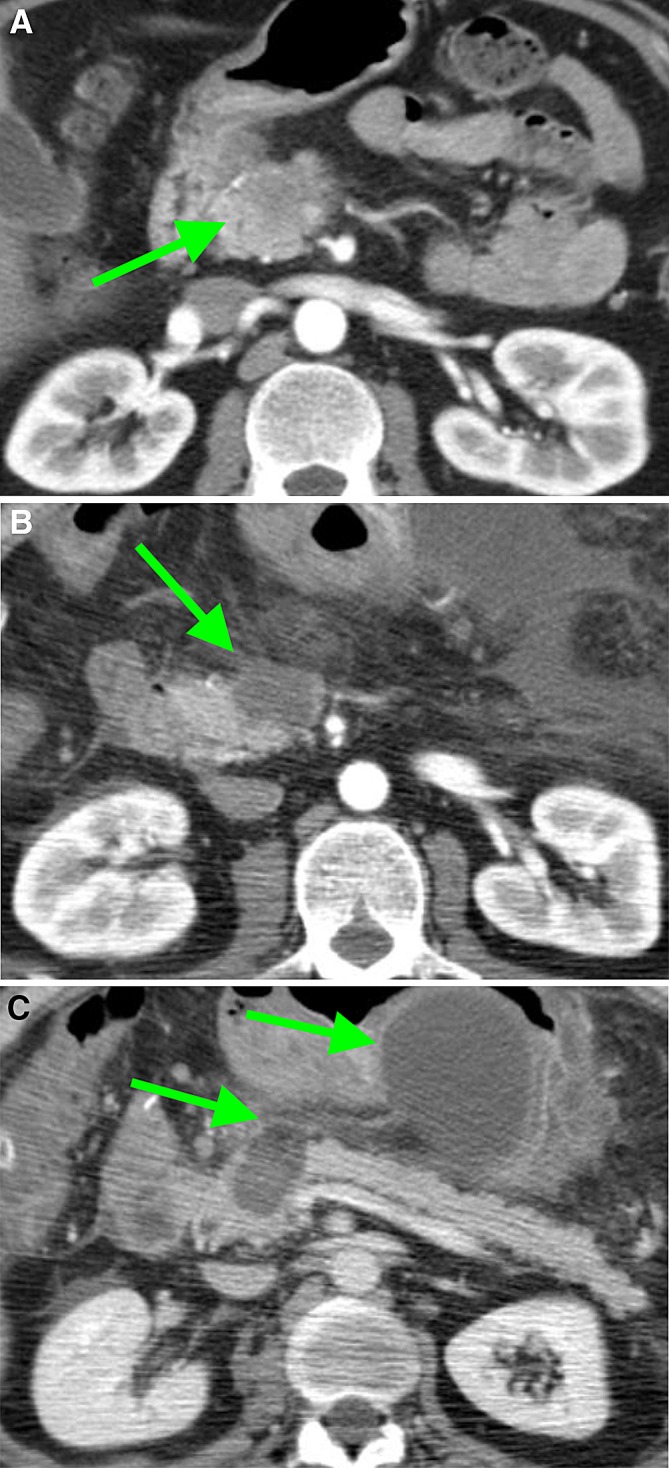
CT-images in the axial plane, of a 56-year-old man with A a ductal adenocarcinoma in the head of the pancreas (arrow) of 3.4 cm transversal on the pre-RFA scan. B and C On CT 1-week post-RFA, the ablation zone included the entire tumor (arrow). C The ablation zone included the ventral contour of the pancreas (left arrow) with an accompanying fluid collection in the lesser sac (right arrow)
